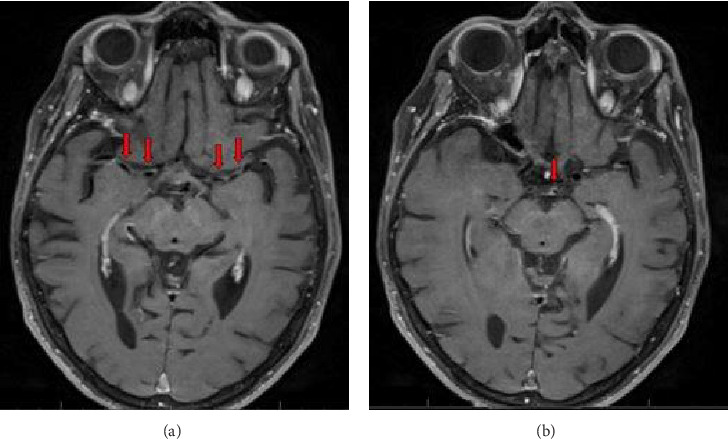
(a) MRI brain T1 post-contrast image demonstrating enhancement of bilateral MCA vessel walls. (b) MRI brain T1 post-contrast image demonstrating enhancement of the basilar artery vessel wall.
